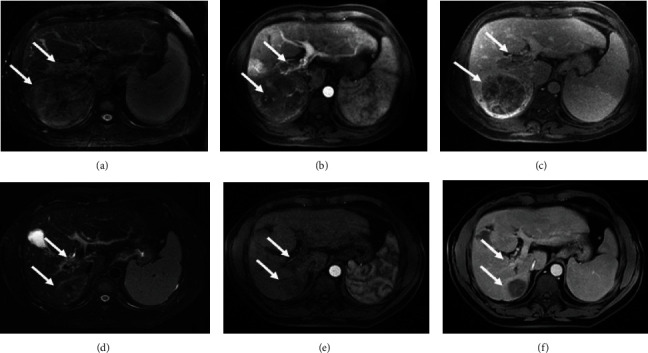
Images from a 45-year-old man with advanced hepatocellular carcinoma (HCC) with Vp3 portal vein tumor thrombosis (PVTT). (a–c) The right main portal vein thrombosis and a huge lesion (84 × 81 mm) in the right lobe of the liver were revealed by MRI T2 phase and contrast-enhanced MRI (CE-MRI) before chemoembolization and hepatic arterial infusion chemotherapy (TACE-HAIC) (arrows). (b–d) After six cycles of TACE-HAIC, MRI T2 phase and CE-MRI imaging showed that the tumor thrombosis and liver lesion were further reduced in size (arrows) with almost no enhancement. The overall survival (OS) was 93.1 months.
